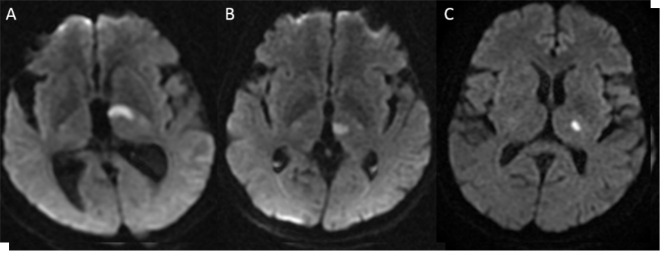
The classic territories of thalamus on DWI: anterior (A), paramedian (B), inferolateral (C). The isolated posterior territory infarction was not observed in present study.
